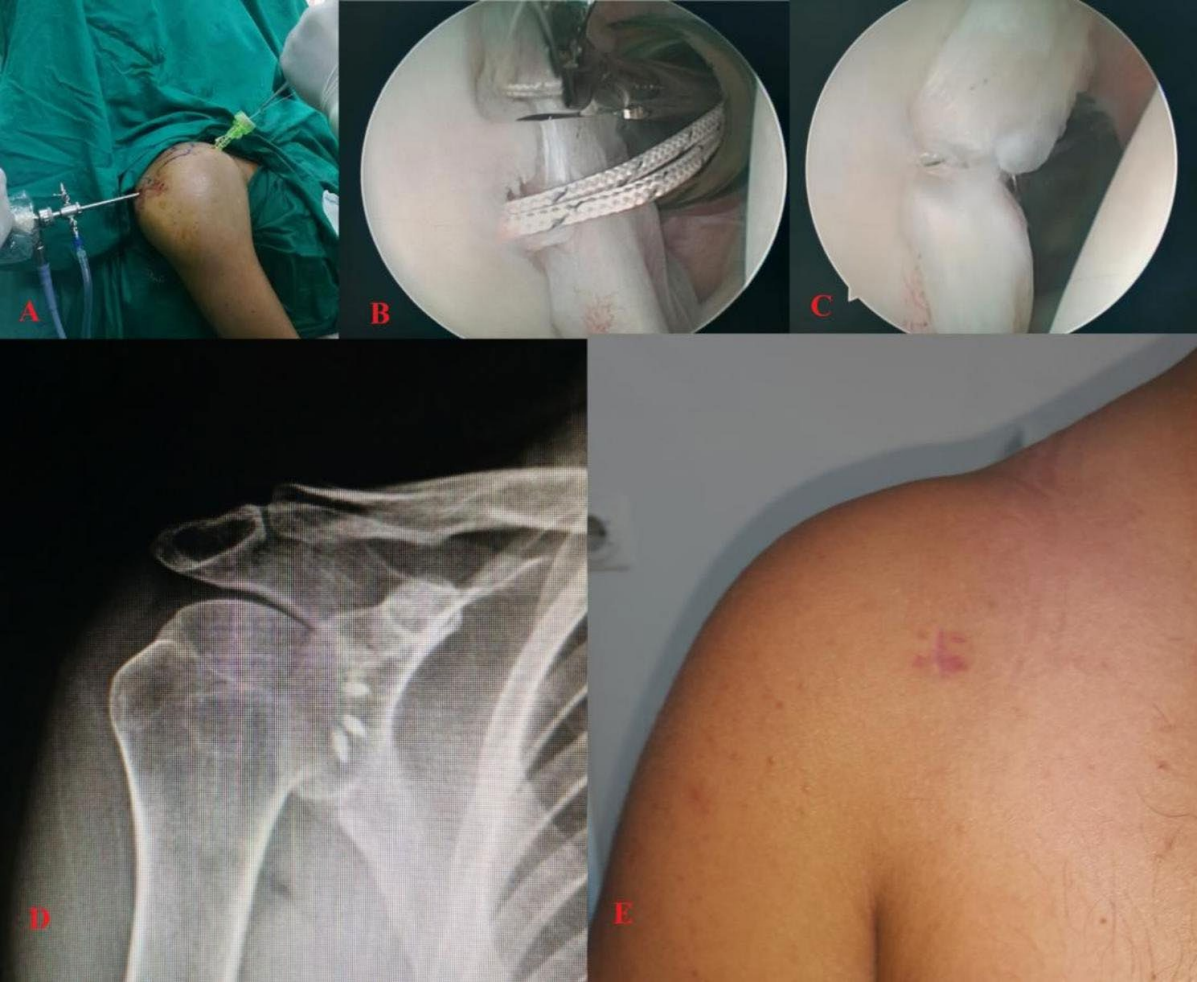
A Shoulder arthroscopy by opening the anterior single portal in the chaise longue position. B View of the birdbeak with threads through one portal after anchor placement. C Labrum after repair. D Postoperative x-ray. E Healed skin incision during control
